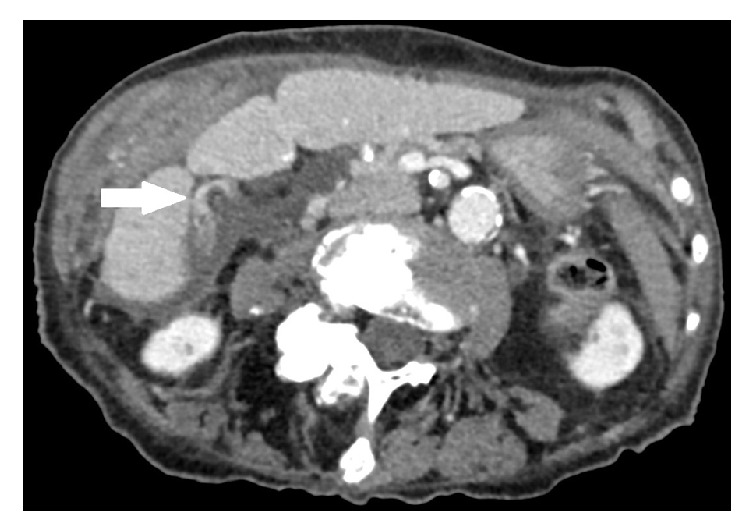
Abdominal contrast enhanced CT scan, portal phase: “Whirl sign” of the gallbladder pedicle.
